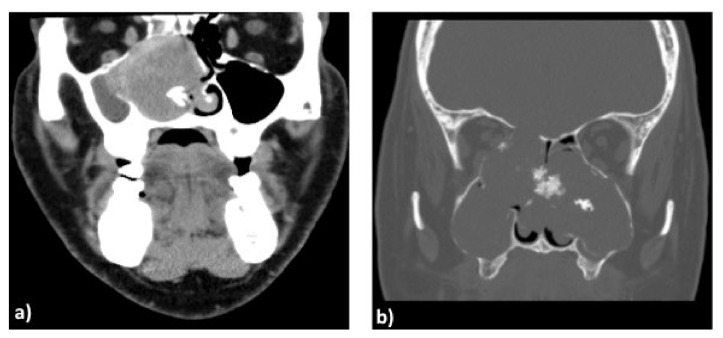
Coronal computed tomography scan showing a large solitary fibrous tumour (SFT) of the right nostril (a) and bilateral chondrosarcoma (b). The hyperostosis of nasal septum indicates the origin of SFT that compresses the medial wall of maxillary sinus, while in (b) it is showed the invasion of maxillary sinus bilaterally.
